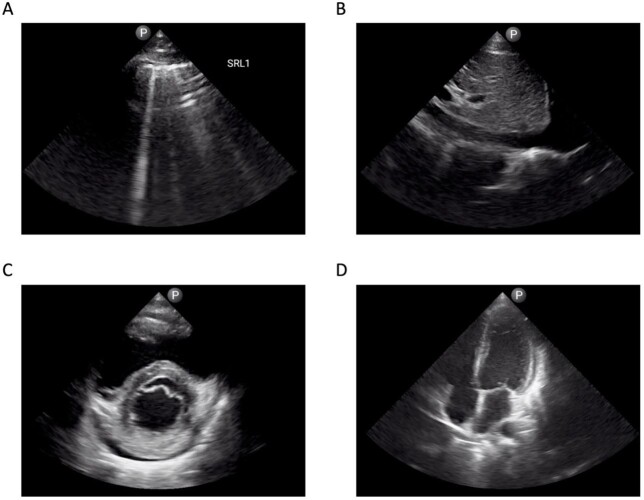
Ultrasound findings from patient 2. 2A: Discrete B lines observed with an irregular pleural line; 2B: Inferior vena cava collapsibility <50% suggestive of adequate fluid resuscitation; 2C: parasternal short cardiac view with small pericardial effusion identified; 2D: apical cardiac view with small pericardial effusion redemonstrated.
